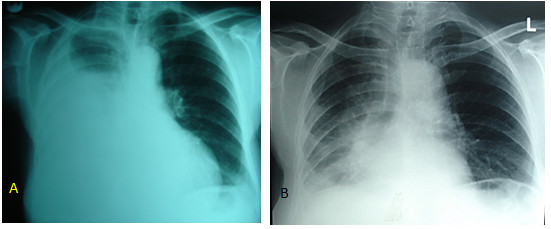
Patient's chest radiograph before (A) and after (B) operation showing massive pleural effusion that is resolved after surgery.
